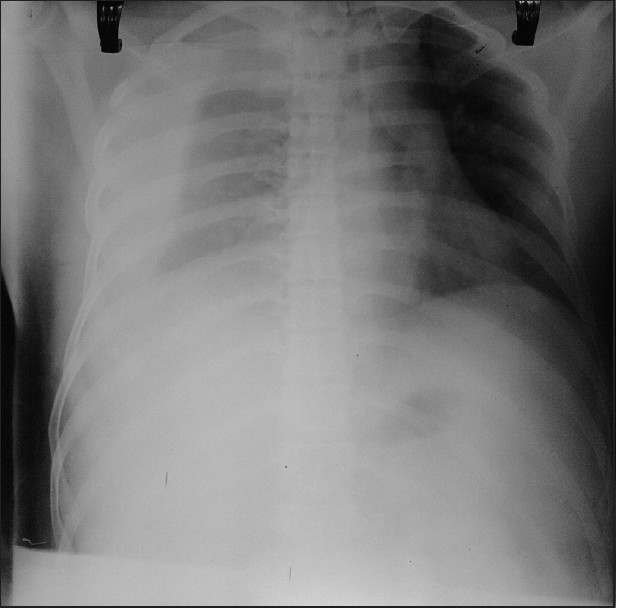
Chest X-ray, haemothorax
